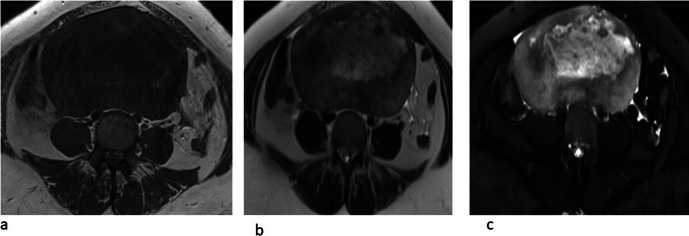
(a, b, c): Axial T1 (5a), T2(5b), and T2FS (5c) MRI images
